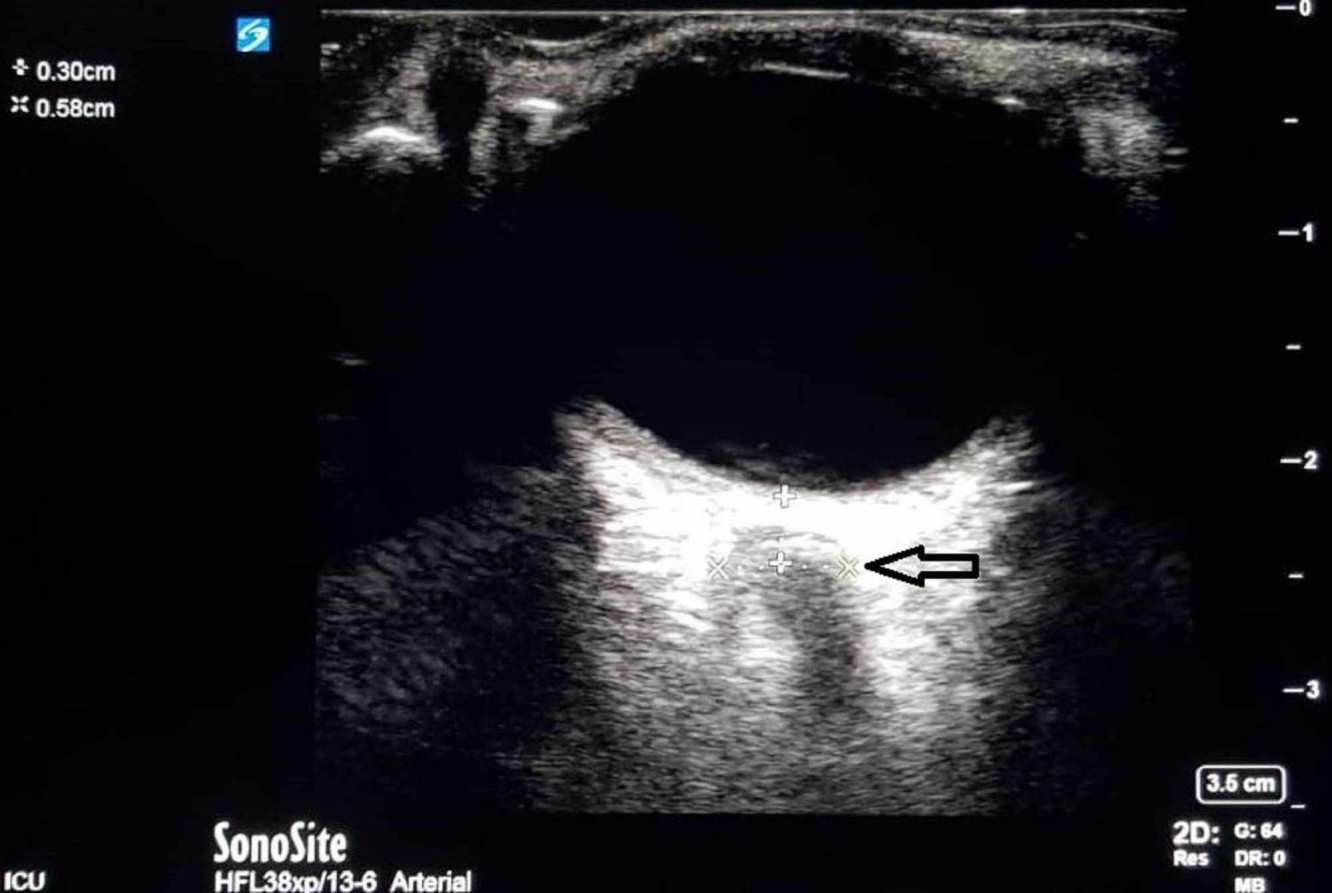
Ultrasound eye performed after 30 h of admission showing optic nerve sheath diameter (distance between two XX) as shown by black arrow.
